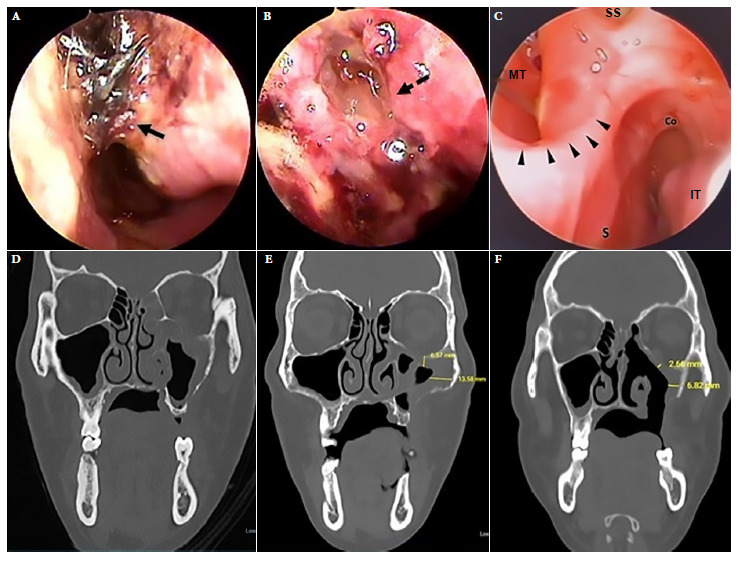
Case 2. Left nasal endoscopy. (A) Endoscopy on admission. (B) Endoscopy during standard treatment. (C) Endoscopy after 30 sessions of treatment with adjuvant hyperbaric oxygen. (D) Facial CT scan. On admission, mucosal hypertrophy of the left maxillary sinus, occupation of anterior ethmoid cells and dehiscence of the orbital floor. (E) CT scan during standard treatment. (F) Tomographic control after 30 sessions of treatment with adjuvant hyperbaric oxygen showing decreased mucosal thickening and a left oroantral fistula. Continuous black arrow: necrotic crusts, dashed black arrow: cerebrospinal fluid fistula, black arrowheads: septal perforation margin. S: septum, IT: inferior turbinate, MT: middle turbinate, SS: sphenoid sinus, Co: choana.
